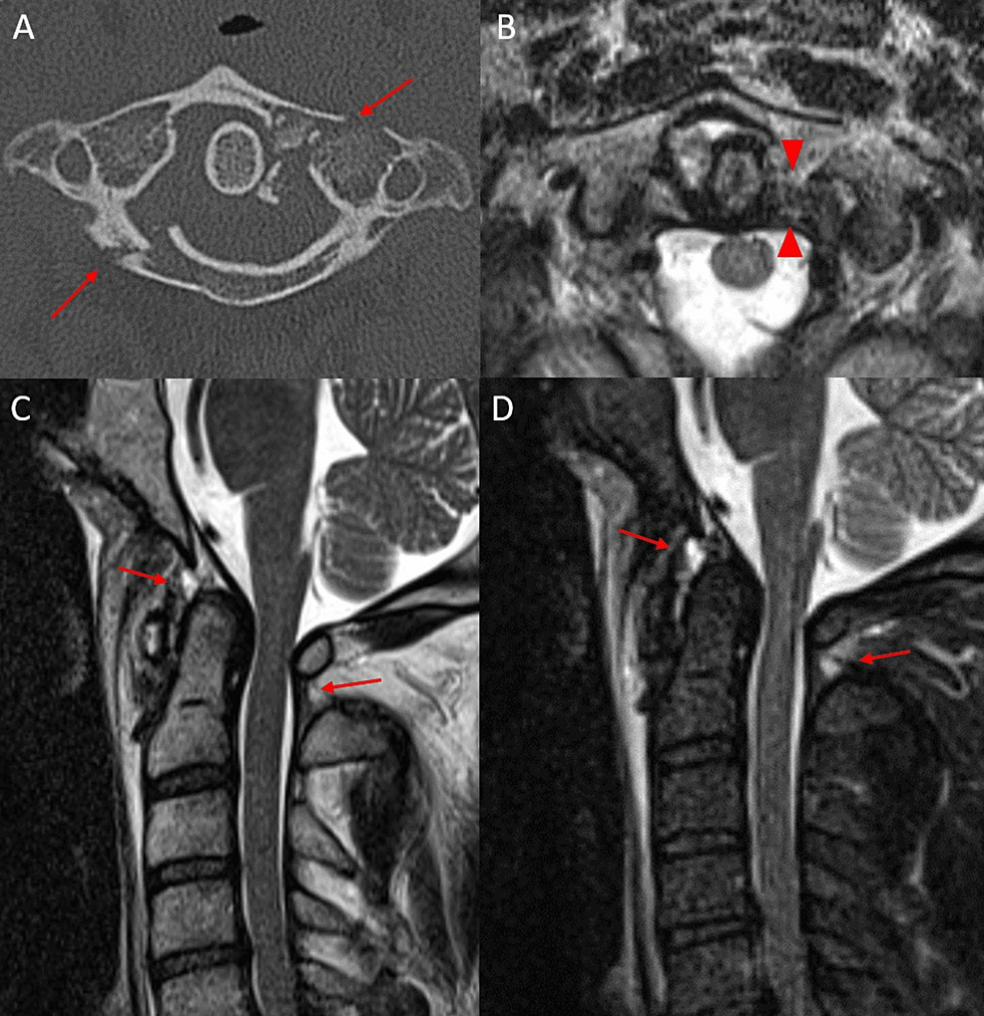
Axial CT of the cervical spine (A) in a 32-year-old trauma patient status post MVA demonstrating comminuted, left C1 anterior and posterior arch fractures (red arrows). Axial T2-weighted MRI (B) in the same patient demonstrating a left transverse atlantal ligament tear (red arrowheads). Sagittal T2-weighted (C) and STIR-weighted (D) MRI of the cervical spine in the same patient demonstrating low-grade anterior and posterior atlanto-occipital membrane complex injuries (red arrows) with otherwise intact major craniocervical junction ligaments. Anterior and posterior atlanto-occipital membrane complex tears were relatively common in the setting of transverse atlantal ligament tears. CT: computed tomography; MVA: motor vehicle accident; MRI: magnetic resonance imaging; STIR: short tau inversion recovery
